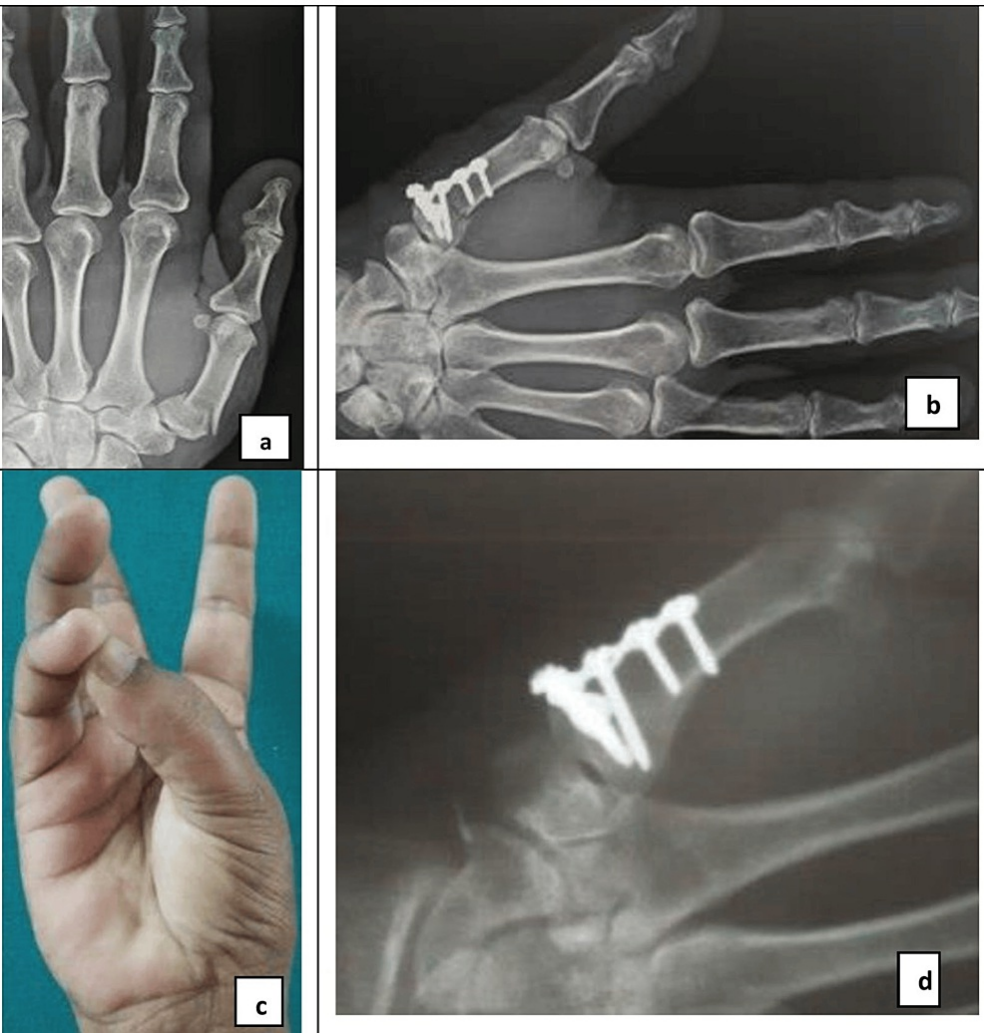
Radiographs showing Oblique fracture of 1st Metacarpal (a), open reduction/Fixation with a T plate (b). Clinical picture showing excellent active range of motion and (c), radiograph showing complete union at fracture site (d).
